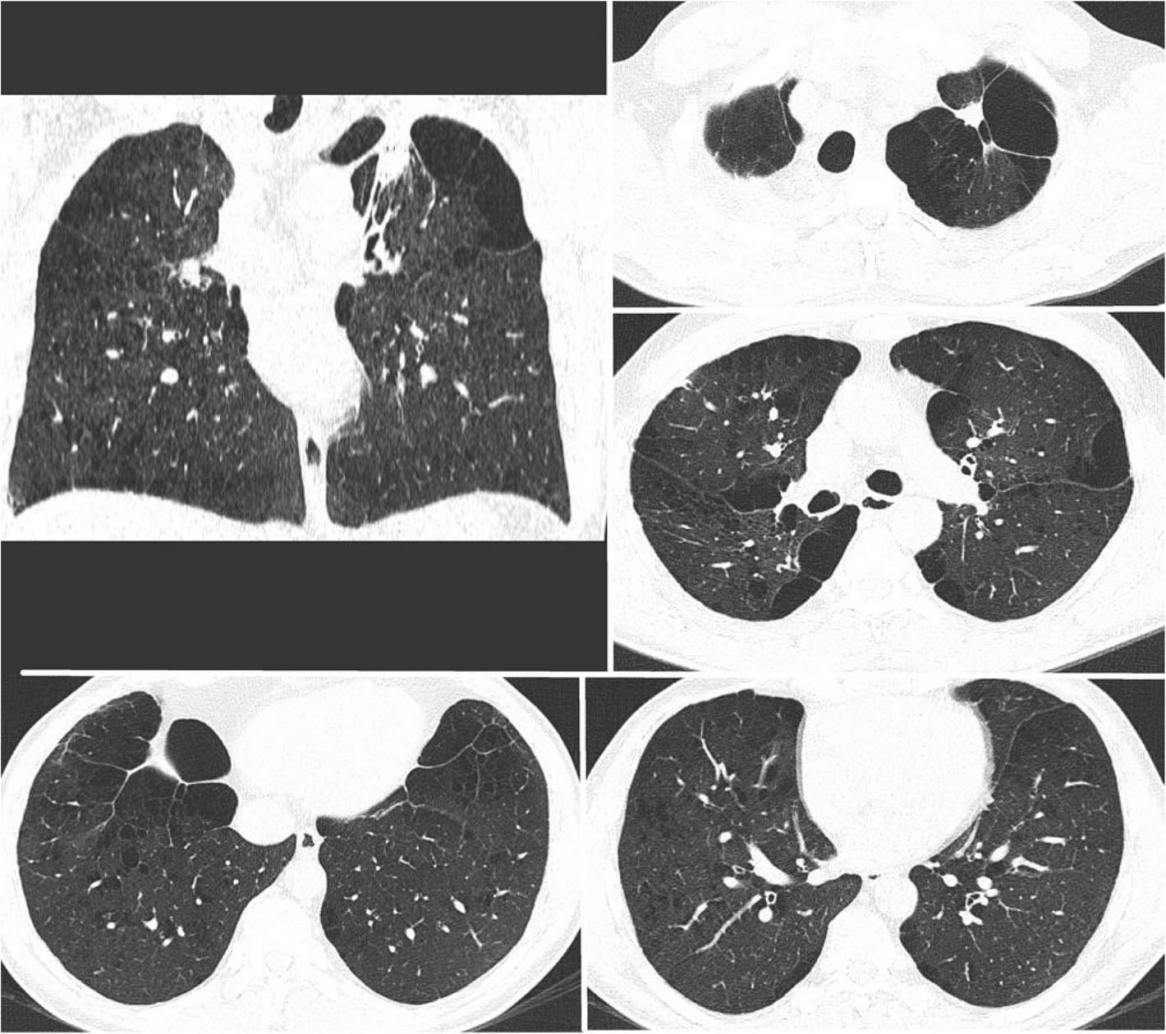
Chest CT scan 6 years past surgery. Both lungs are extended and fulfills hemithorax volume. In upper and middle sections of both lungs bullae up to 6 cm in diameter are visualized
